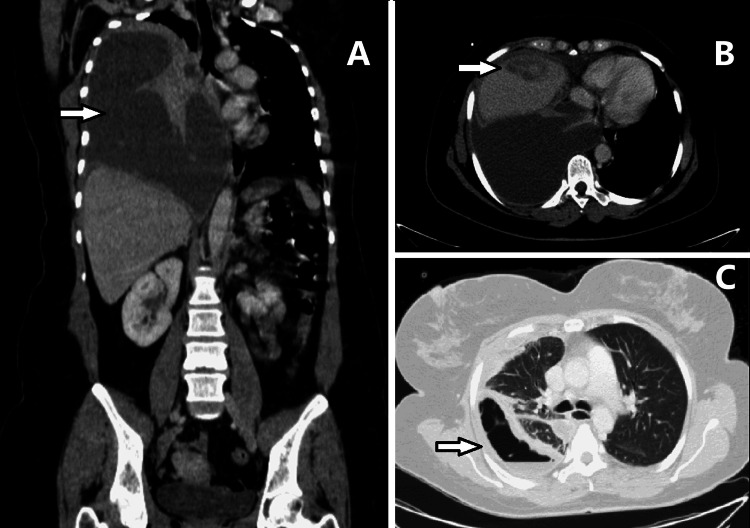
Computed tomography (CT) scan showing a pyogenic liver abscess complicated with pulmonary involvement (A) Coronal view of the thoracoabdominopelvic CT scan showing a massive right pleural effusion, with a partially loculated appearance and enhancement of the pleural leaflets, suggestive of empyema (arrow), causing a shift to the left side of the mediastinal structures; (B) Axial view of the thoracoabdominopelvic CT scan showing multiloculated empyema in the right pleural cavity. In the upper segments of the right hepatic lobe, there remained a heterogeneous area with a hypodense center concerning the known liver abscess (arrow) with a dimensional reduction, maintaining the apparent contiguity of the liver abscess with the adjacent pleural space; (C) Axial view of the thoracic CT scan showing right lung entrapment (arrow).
